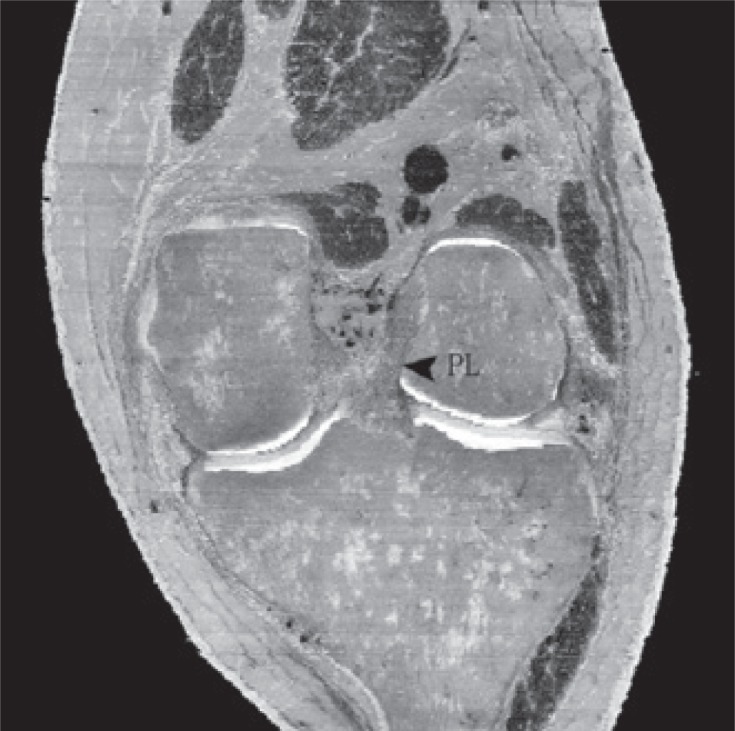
Oblique coronal plane magnetic resonance imaging (MRI) showing the anteromedial (AM) bundle bundle (arrow).
